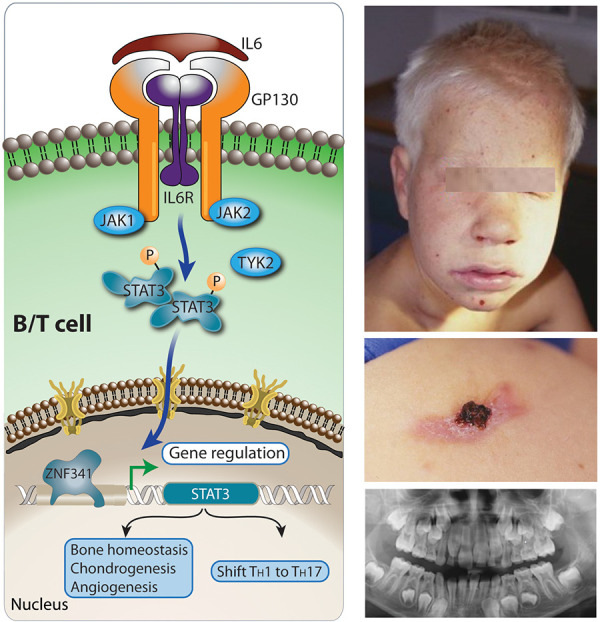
Physiology of STAT3 signaling (left panel) and a 7-year-old boy with coarse facial features (right panel, above), eczema, cold skin abscesses (middle) and delayed dentition at 10 years of age (below, different patient). IL6 signals through the IL6RST (signal transducer of IL6 receptor complex), composed of glycoprotein 130 (GP130) and the IL6R. Other cytokines of the IL6 cytokine family utilize GP130 encoded by *IL6ST* (IL11, OSM, LIF, CNTF, CT-1, CLCF1, IL27, IL35, and IL39). IL6 then activates through JAK-STAT signal transduction the zinc finger protein 341 (ZNF341) TF, which promotes STAT3 transcription. IL6 and IL23 activate *STAT3*, which turns on *RORC*, encoding RORγt the master regulator for a TH1/TH17 T-cell response shift as well as chondrogenesis, bone repair, angiogenesis/vascularization (induction of vascular endothelial factor 3 (VEGF3)) [23]. The phosphoglucomutase 3 (PGM3) (not shown in figure) enzyme catalyzing the isomerization of N-acetyl-glucosamine-6-phosphate to N-acetyl-glucosamine-1-phosphate during the generation of UDP-N-acetyl-glucosamine UDP-GlcNAc. During glycosylation, sugar chains are added to either proteins or lipids, using basic sugar building blocks (UDP-GlcNAcs) to make N-glycans, O-glycans, proteoglycans, and glycosylphosphatidylinositol (GPI)-anchored proteins all participating in cell signaling.
